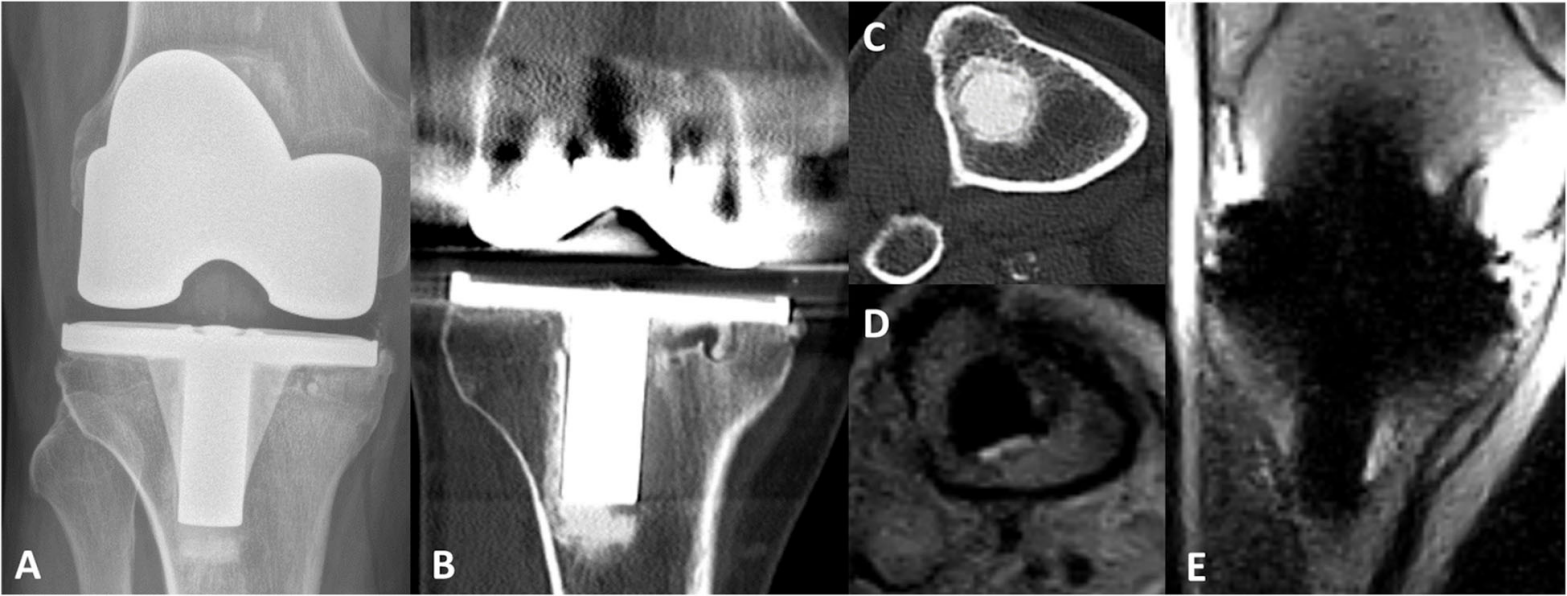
Loosening of the tibial component. The conventional radiograph (a) of patient 6 shows tibial component loosening around the medial plate and stem. CT (b and c) and low-field MRI (d and e) show images of the same knee, where images c and d are the transversal views of b and e at the most distial point of the tibial stem. The CT shows lucency (b-c) and MRI effusion (d-e) around the tibial stem, which are elements suspected of loosening the tibial component
